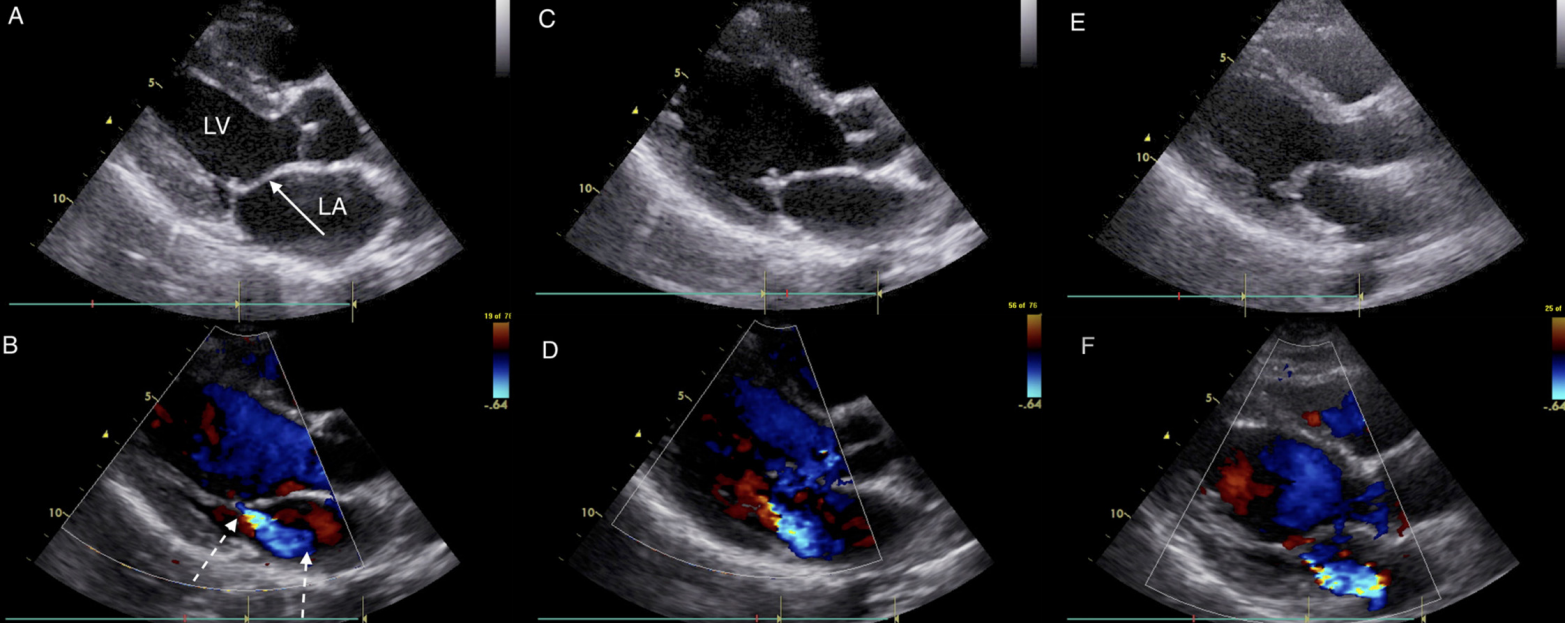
Images from a family with 3 RHD positive members: Index Case, Sibling and Mother. Parasternal long axis echocardiographic still frames in early systole in black and white (panels A, C, and E) and color Doppler (panels B, D, and F) of RHD positive index case (panels A and B), sibling (panels C and D) and mother panels (E and F). The black and white panels all show a thickened anterior mitral valve leaflet (solid arrow) and prolapse (excessive tip motion) of tip of anterior mitral valve leaflet. The mother has the most extreme thickening of the valve along with thickened chordae and immobile valve motion (not seen on these frames). The color Doppler panels show similar mitral regurgitation jets (dotted arrows) measuring approximately 3 cm in each subject. Both RHD positive index case and sibling also had aortic insufficiency jet measuring > 1 cm (not shown). Abbreviations: LA–left atrium, LV–left ventricle.
